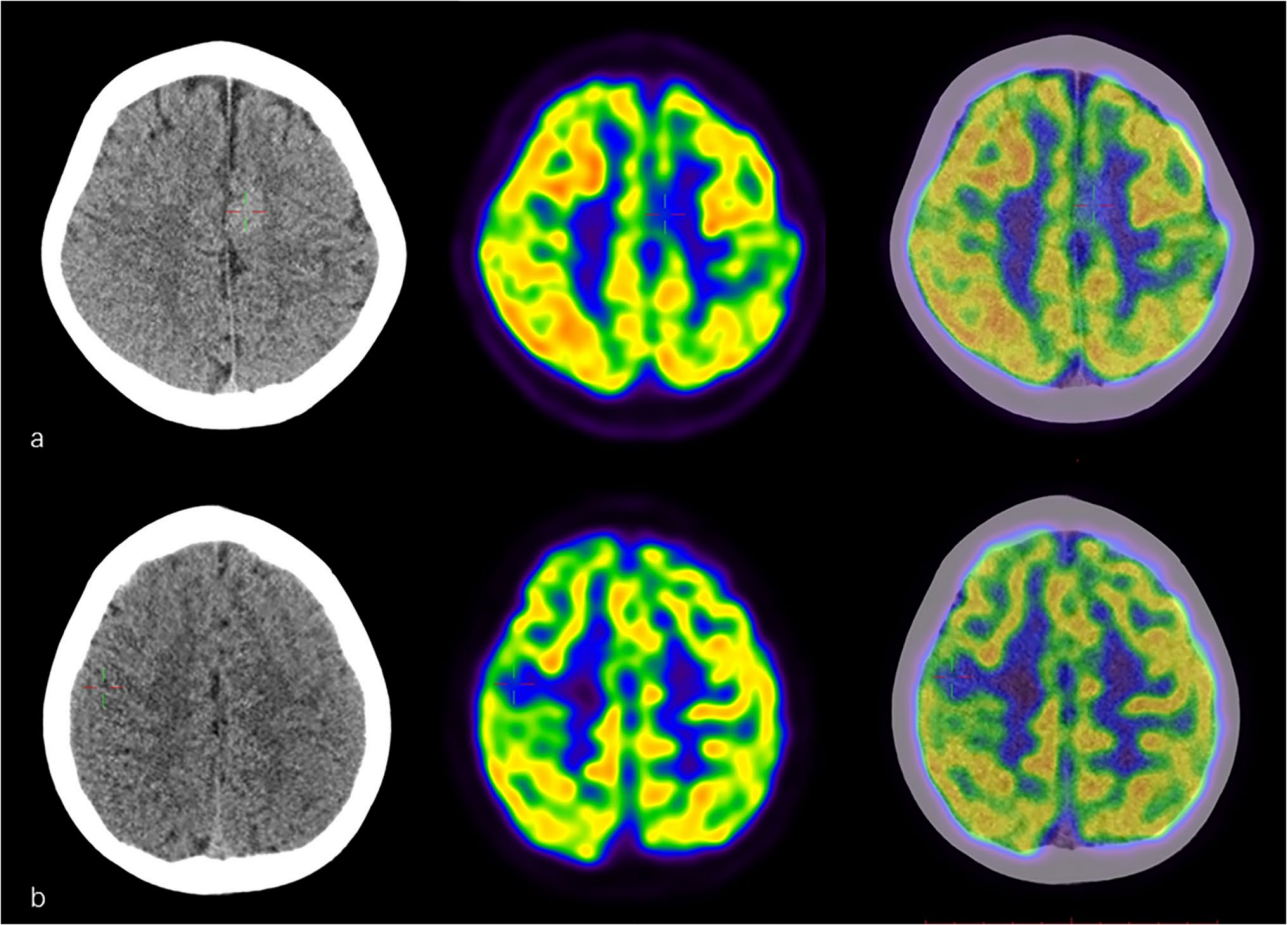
a A 6-year-old boy with a 9-month history of seizures. Brain ^18^F-FDG PET/CT showed focal hypometabolism in left frontal lobe (the cross) with high density on CT correspondingly (the cross). The boy was finally diagnosed with frontal epilepsy. b A 6-year-old girl with a 4-month history of seizures. Brain ^18^F-FDG PET/CT indicated focal hypometabolism in right central sulcus mainly in the parietal lobe (the cross) with no abnormality found on CT correspondingly (the cross). The girl got well recovery after immunotherapy and was finally diagnosed with seronegative AE
